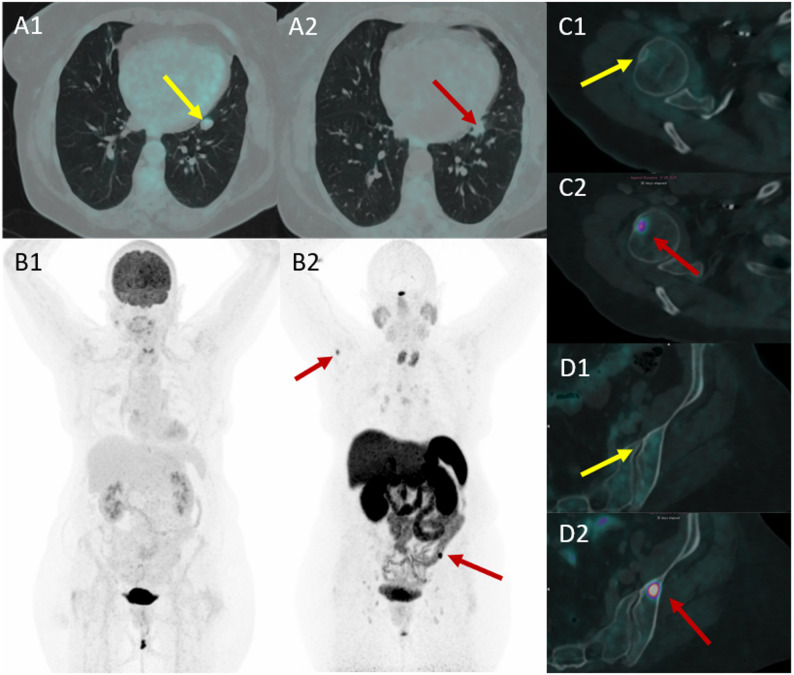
66-year-old woman after resection of a lobular carcinoma of the right breast. [¹⁸F]FDG PET/CT (A1) - axial fused image, the paracardial lesion (yellow arrow) has low metabolic activity. Biopsy showed a typical carcinoid (NET G1, Ki-67 5%, Estrogen receptor-negative). For staging of the pulmonary carcinoid, a [⁶⁸Ga]Ga-DOTATATE PET/CT was performed and the axial fused image (A2) showed only faint somatostatin receptor 2 (SSTR2) expression (red arrow). Whole-body maximum intensity projection of [¹⁸F]FDG PET/CT (B1) shows no increased uptake in the bones. However, on [⁶⁸Ga]Ga-DOTATATE PET/CT (B2) two bone lesions with intense [⁶⁸Ga]Ga-DOTATATE uptake were detected (red arrows). On axial fused [¹⁸F]FDG PET/CT (C1/D1) sclerotic changes, without [¹⁸F]FDG uptake were seen (yellow arrow), where fused [⁶⁸Ga]Ga-DOTATATE PET/CT showed strong SSTR2 expression (SUVmax 10.2) (red arrow) in the right humeral head lesion (C2) as well as in the left iliac lesion (D2). The iliac bone lesion was biopsied and a SSTR2 positive bone metastases of the lobular breast cancer confirmed
